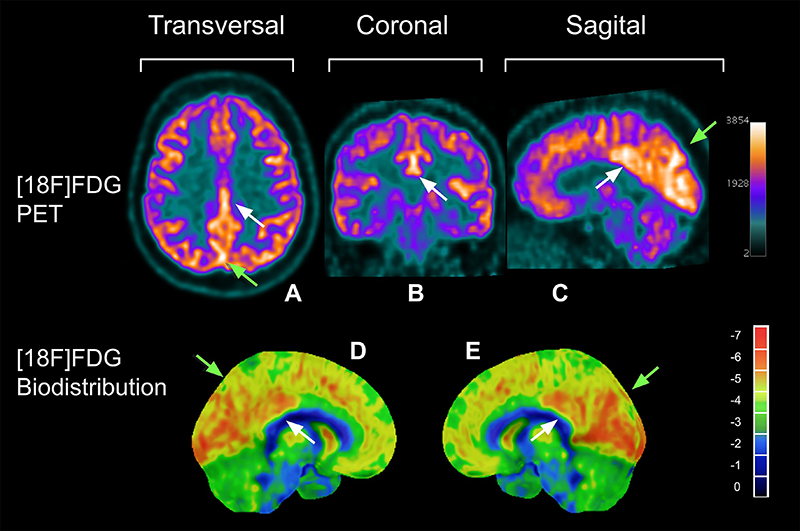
A, B, and C, transversal, coronal, and sagittal brain views by [18F]FDG PET of a patient with glycolytic hypermetabolism. D and E, bilateral mesial aspects of a 3D exhibition of the biodistribution of the radiopharmaceutical on the brain via CortexID^©^ software (General Electric). White arrows indicate the cerebral cortex with glycolytic hypermetabolism on the posterior cingulate, and green arrows indicate the precuneus parietal regions. FDG: fluorodeoxyglucose; PET: positron emission tomography.
